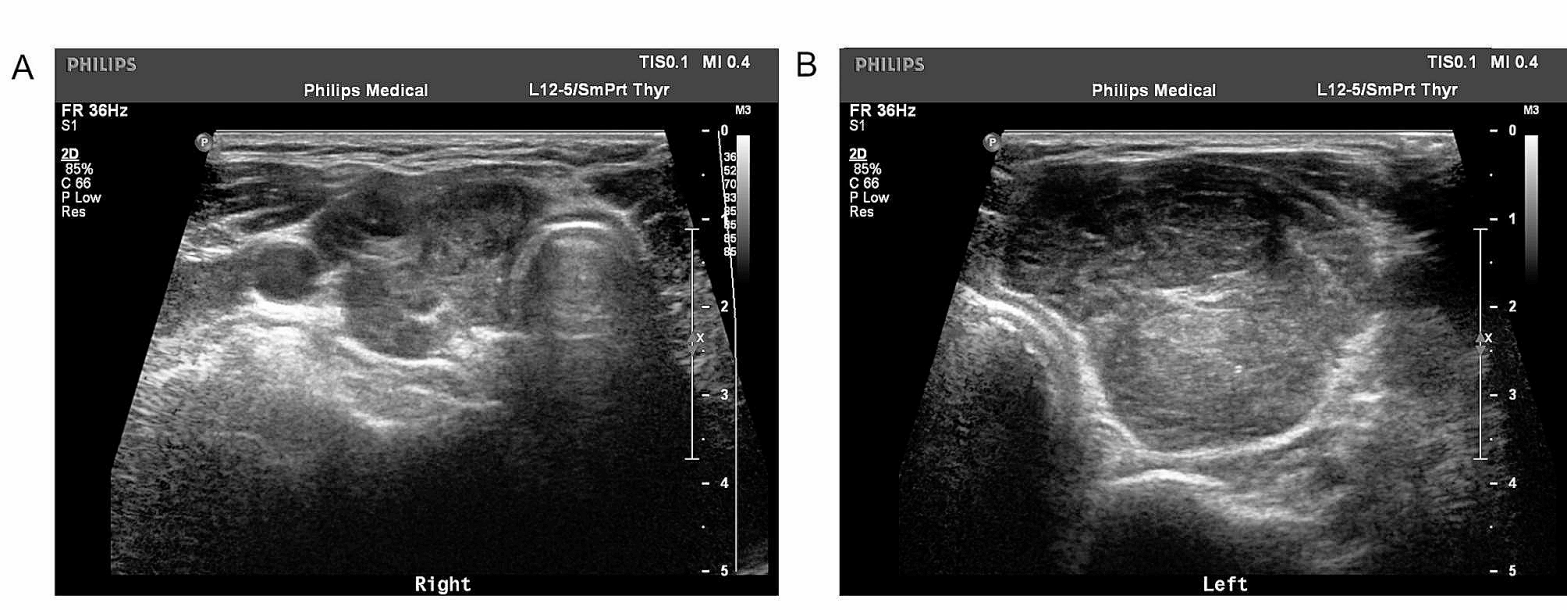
The transverse section of the thyroid from a type-B primary thyroid lymphoma patient. The anterior-posterior diameter was 2.2 cm for the right lobe (A), and 3.4 cm for the left lobe (B). In addition, sonographic features of strip echo and grid echo were present for this patient
